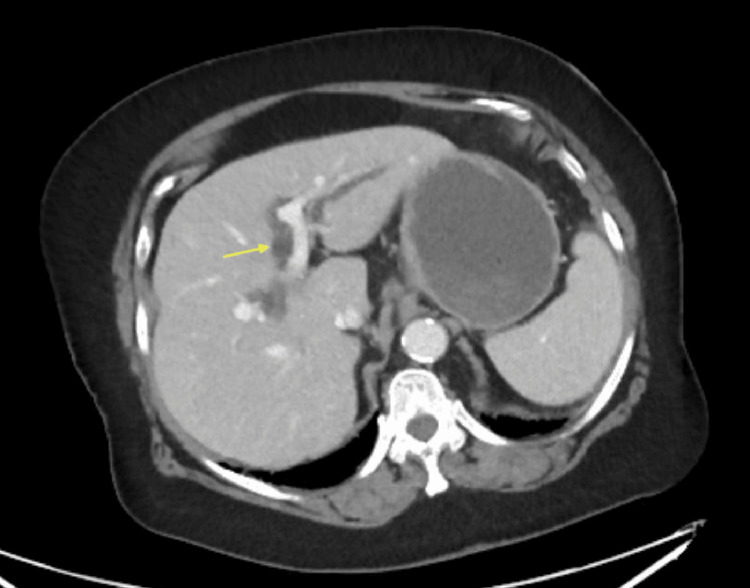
Axial abdominal CT scan showing intrahepatic bile duct dilated to >2.5 mm suggestive of biliary obstruction (yellow arrow) CT: computed tomography
